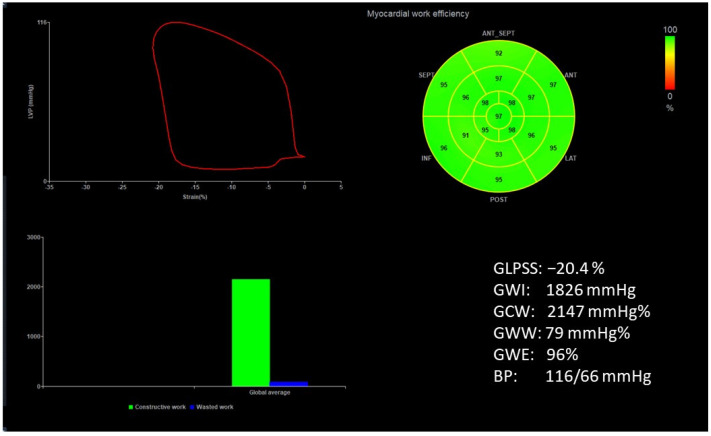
An example of echocardiography of a 30-year-old healthy male. A set of individual longitudinal strain curves for all segments shown in different colors, with the average curve shown as a white dotted line, a bull’s eye of segmental longitudinal peak systolic strains and a summary of global longitudinal peak systolic strain (GLPSS) are shown in the top panel. The LV pressure–strain loop with the bull’s eye representation of myocardial work efficiency for each of the segments and a summary of all myocardial work indices are shown in the lower panel. Abbreviations: GLPSS—global longitudinal peak systolic strain, EF—ejection fraction, GWI—global myocardial work index, GCW—global constructive work, GWW—global wasted work, GWE—global work efficiency, BP—blood pressure.
